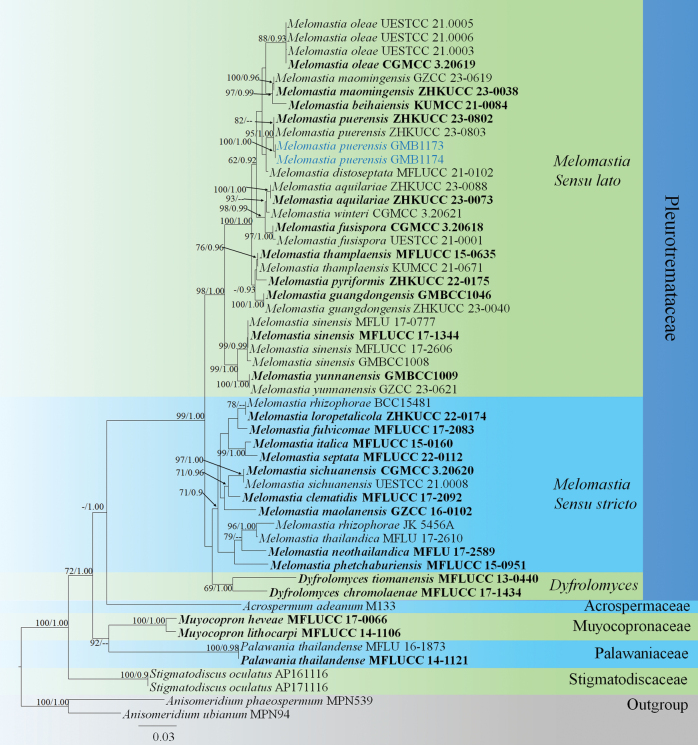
RAxML tree based on a combined dataset of LSU+SSU+*tef*1-α gene sequences. The topology of the trees generated by both maximum likelihood (ML) and Bayesian inference (BI) analyses exhibited high similarity. The RAxML tree with a final ML optimization likelihood value of -12726.117171. The aligned matrix had 933 distinct alignment patterns, with 22.57% of undetermined characters or gaps. Parameters for the GTR+I+G model of the combined LSU, *tef*1-α, and the SYM +I+G model of the combined SSU were as follows: estimated base frequencies A = 0.239385, C = 0.263871, G = 0.289634, T = 0.207110; substitution rates AC = 0.851550, AG = 2.071762, AT = 1.124966, CG = 0.971448, CT = 7.978925, GT = 1.000000; the proportion of invariable sites I = 0.478880; and gamma distribution shape parameter *α* = 0.661129. Bootstrap support values for ML equal to or greater than 60% and PP equal to or greater than 0.90 are given above the nodes. New records are in blue, while the ex-type species are in bold.
